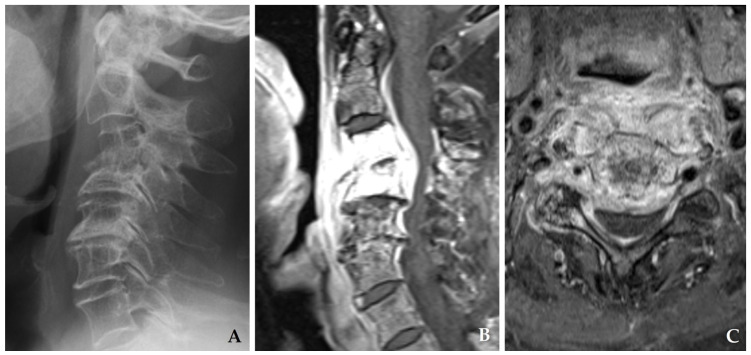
Plain radiograph (A) and magnetic resonance imaging (B,C) demonstrate destructive changes in the C3–C4 vertebral bodies with kyphotic deformity accompanied by retropharyngeal and epidural abscesses compressing the spinal cord.
